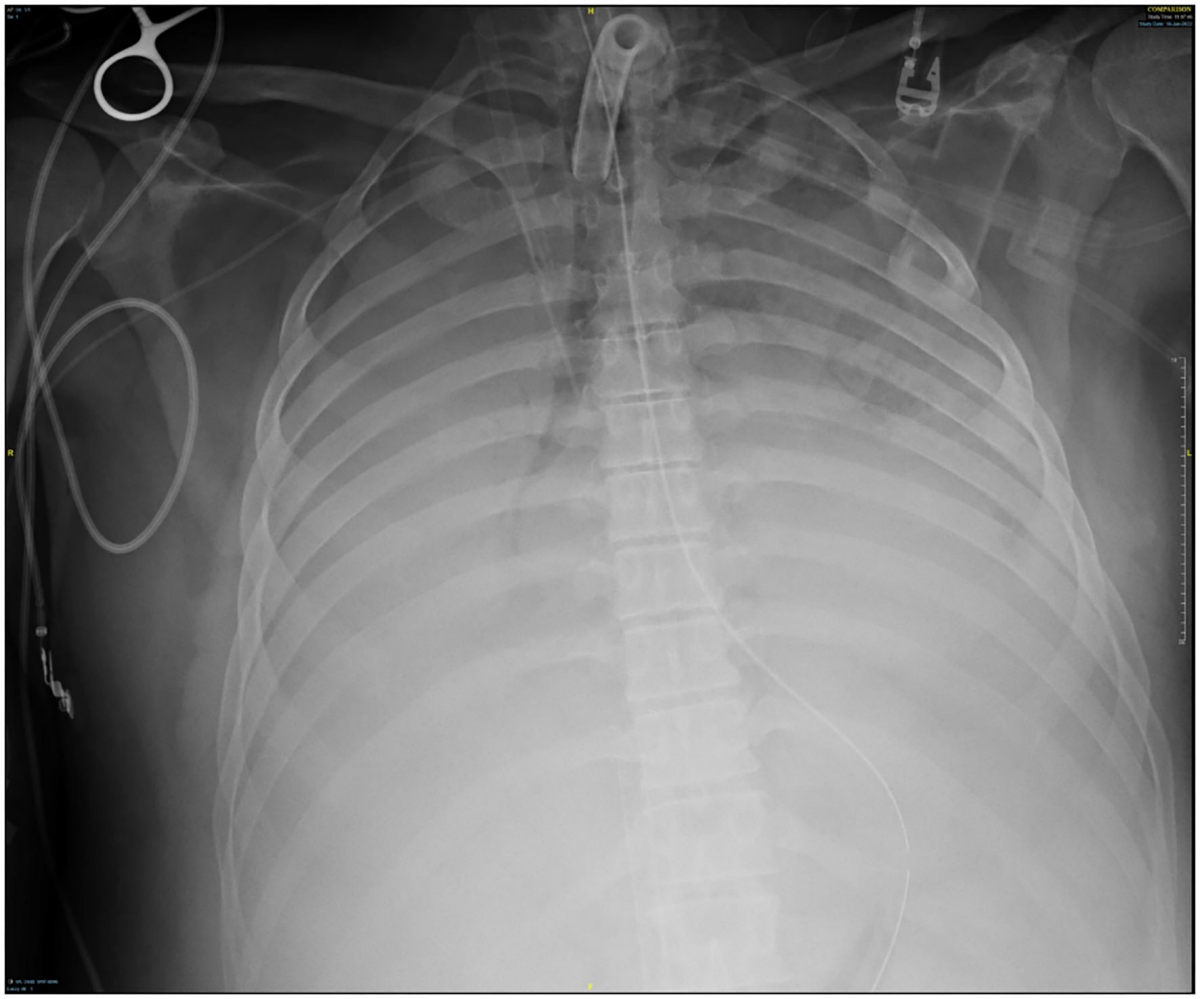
Chest x-ray 2 days after resuming ventilator.
